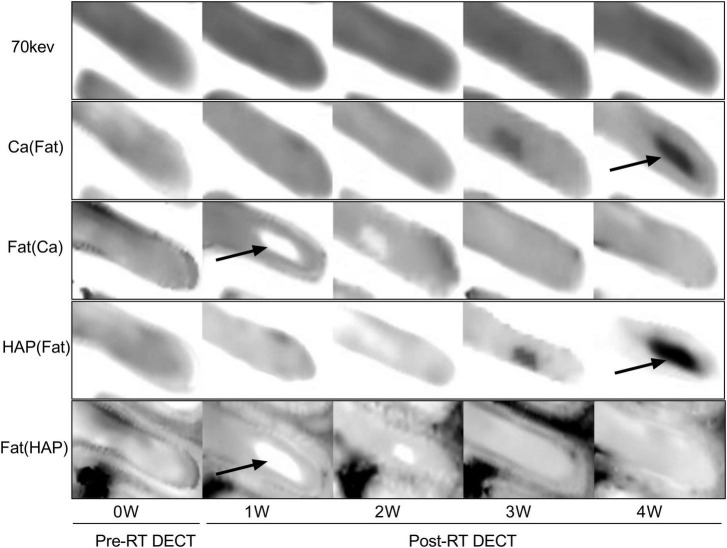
Imaging analysis of radiotherapy effects on the right femoral neck. Representative conventional single-energy CT (70 keV) images and corresponding DECT material decomposition maps: Ca-based MD images [Ca (Fat) and HAP (Fat)] and fat-based MD images [Fat (Ca) and Fat (HAP)] at different post-radiotherapy intervals.
